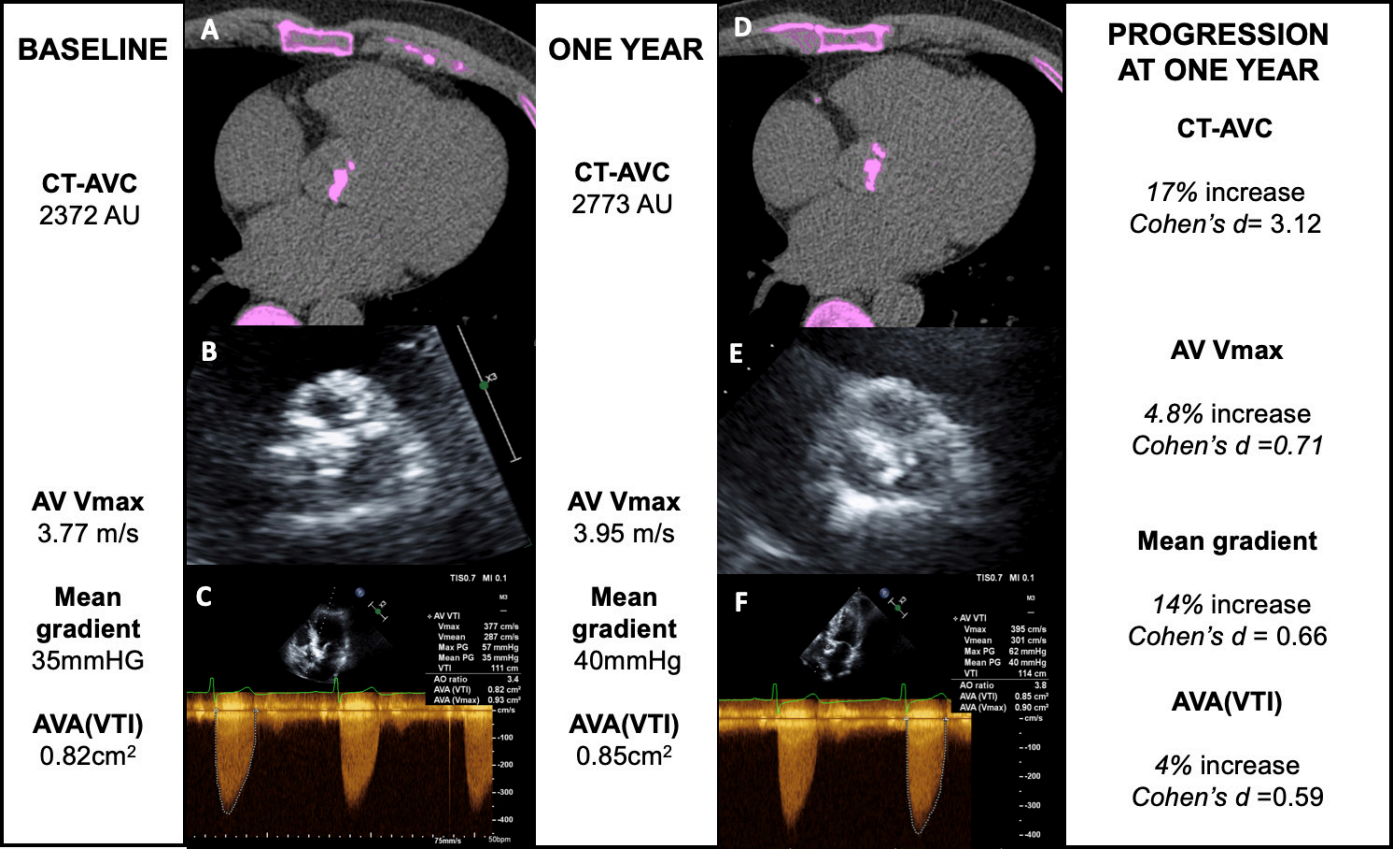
CT calcium scoring and echocardiography to monitor disease progression in aortic stenosis. CT calcium scoring of the aortic valve (AV) and echocardiography in a patient at baseline (A–C) and 1 year (D–F). Baseline CT calcium scoring demonstrates CT quantification of aortic valve calcification (CT-AVC) of 2372 AU (A), transthoracic echocardiography of the AV shows calcified leaflets with a calcium score of 4 (B) and Doppler echocardiography demonstrates a peak velocity of 3.77 m/s (C) at baseline. At 1 year, CT-AVC has increased to 2773 (D), the AV calcium score on echocardiography is graded as 4 (E) and the peak jet velocity has increased to 3.95 m/s (F). AVA, aortic valve area; VTI, velocity time integral.
